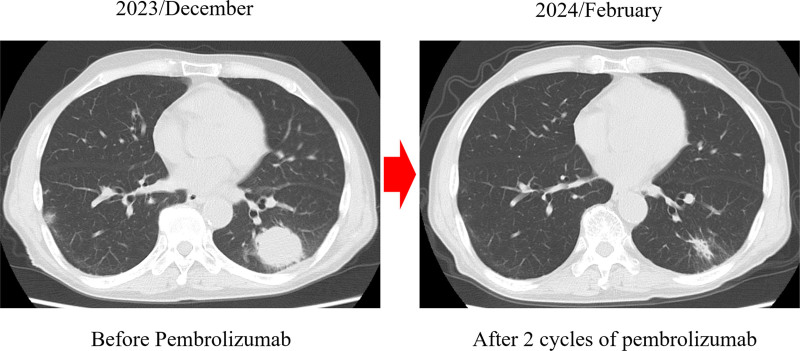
Computed tomography after pembrolizumab monotherapy. The tumor shrunk after 2 cycles, and the patient achieved a PR. PR = partial response.
